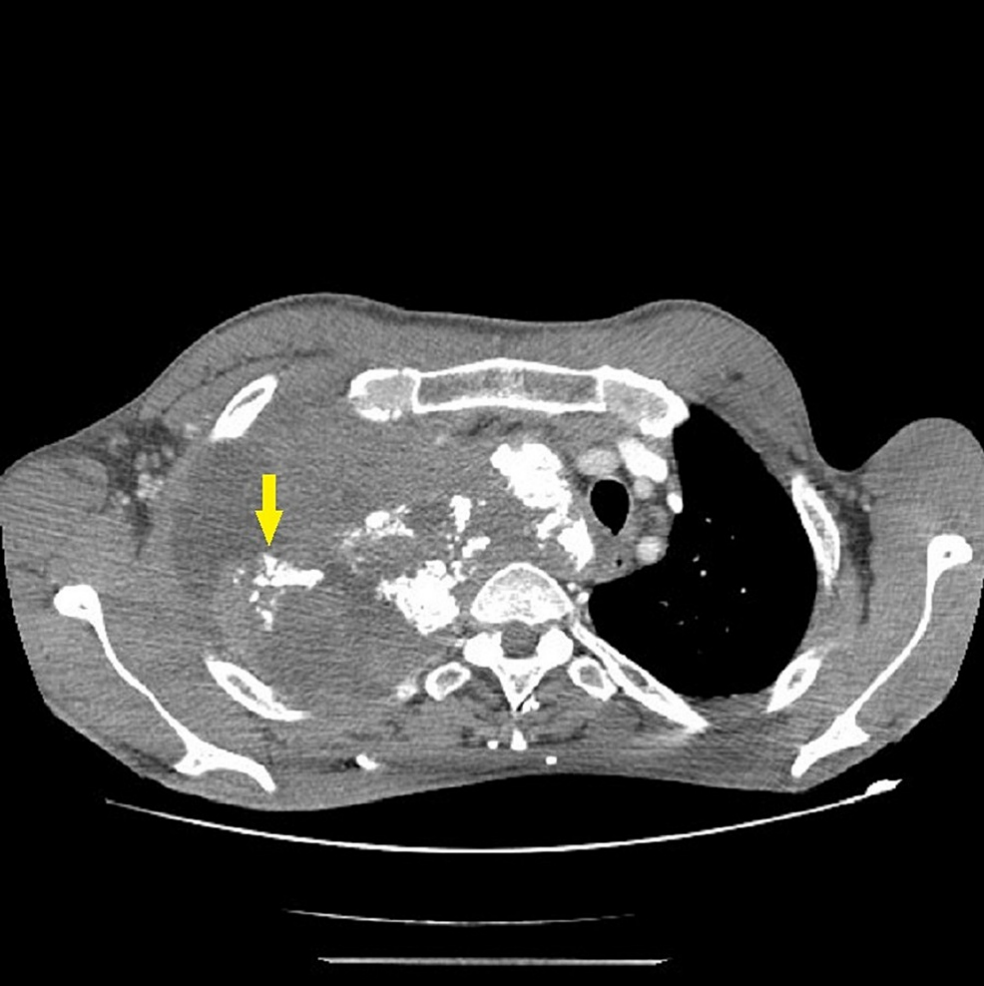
CT scan showing the tumor containing calcifications and invading of mediastinum
